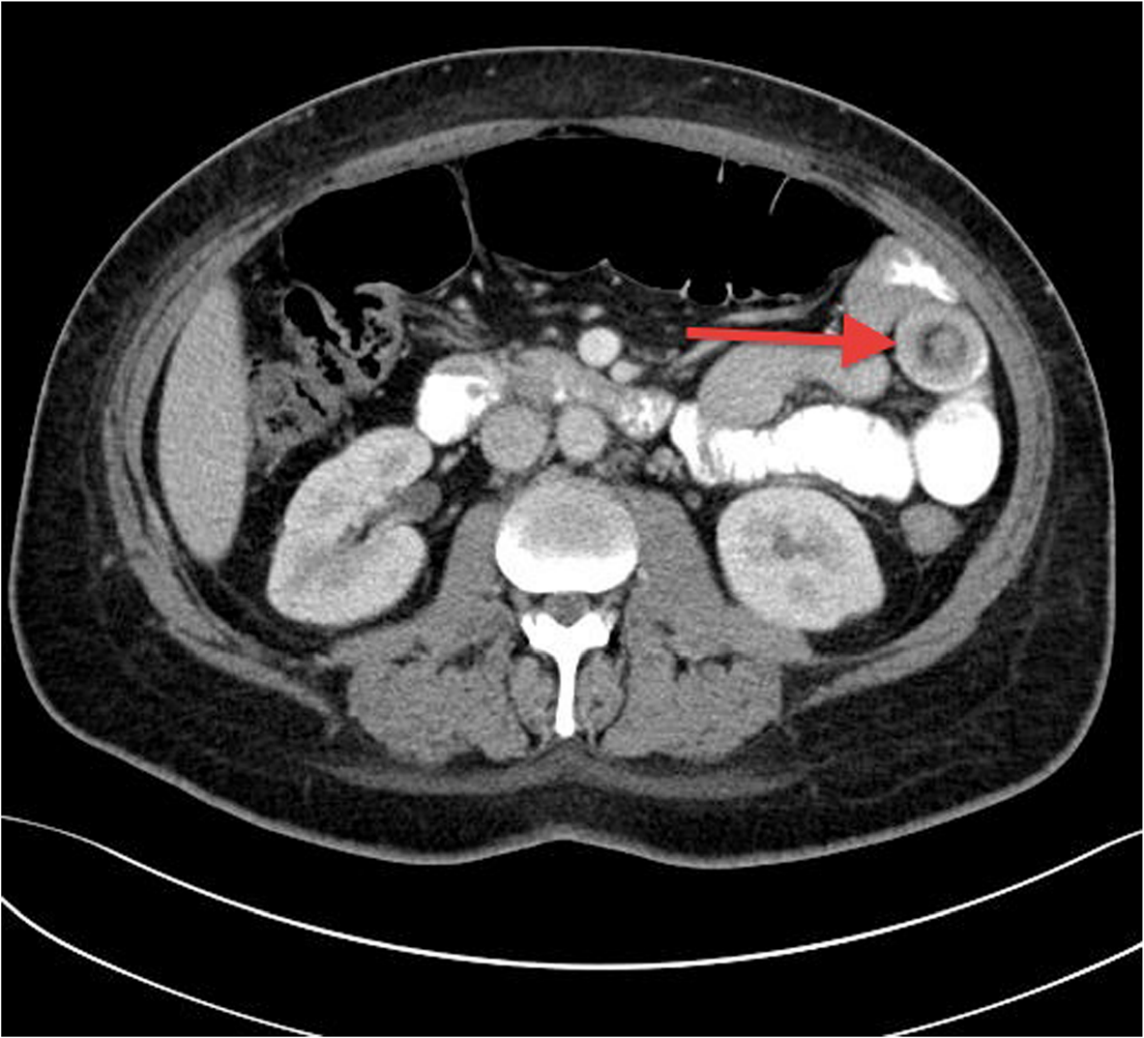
A CT scan of the abdomen (transverse section); showing small bowel loops filled with oral contrast and a target sign appearance at the left side of the abdomen (arrow).
